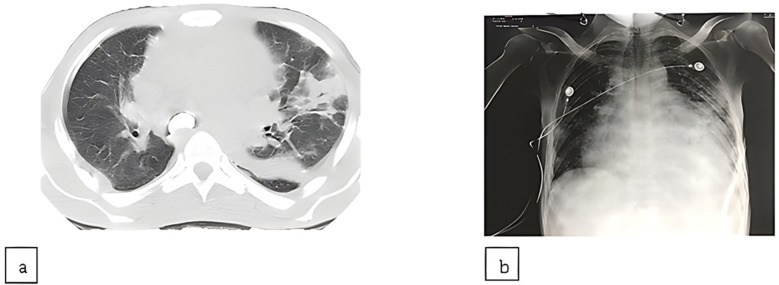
(a) Computed tomography (CT), (b) Chest X-ray chest images without contrast.
